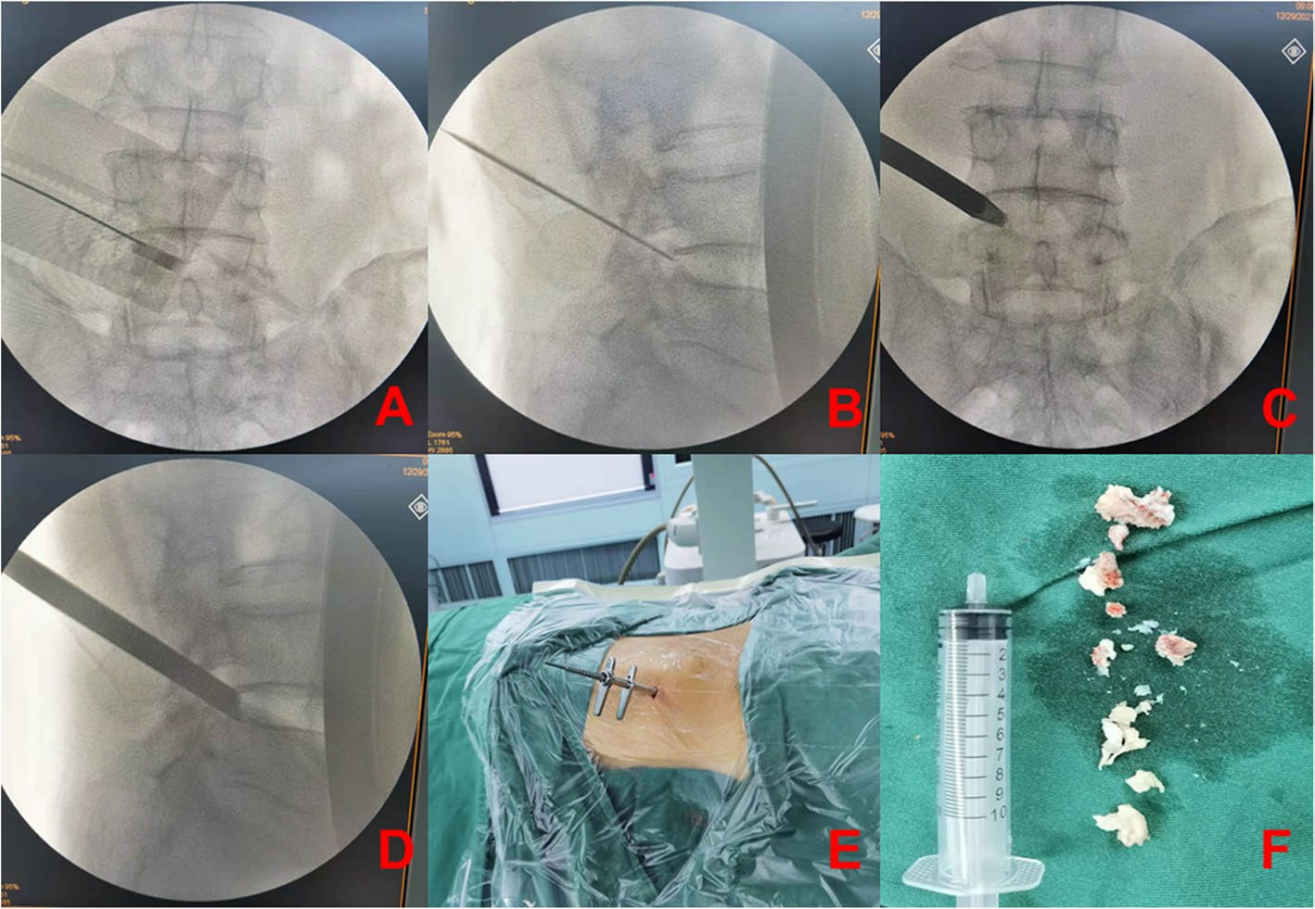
The PELD working channel in place to complete the surgery. A-E Place the cannula step by step after confirming the correct position under X-ray fluoroscopy. F Lumbar 4/5 herniated disc tissue was removed during surgery
